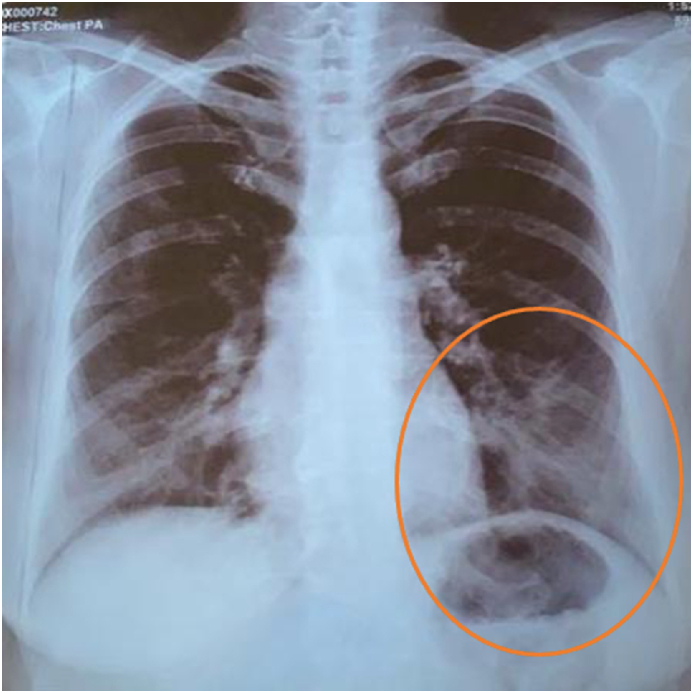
Chest X-Ray (april 2nd,2022).
